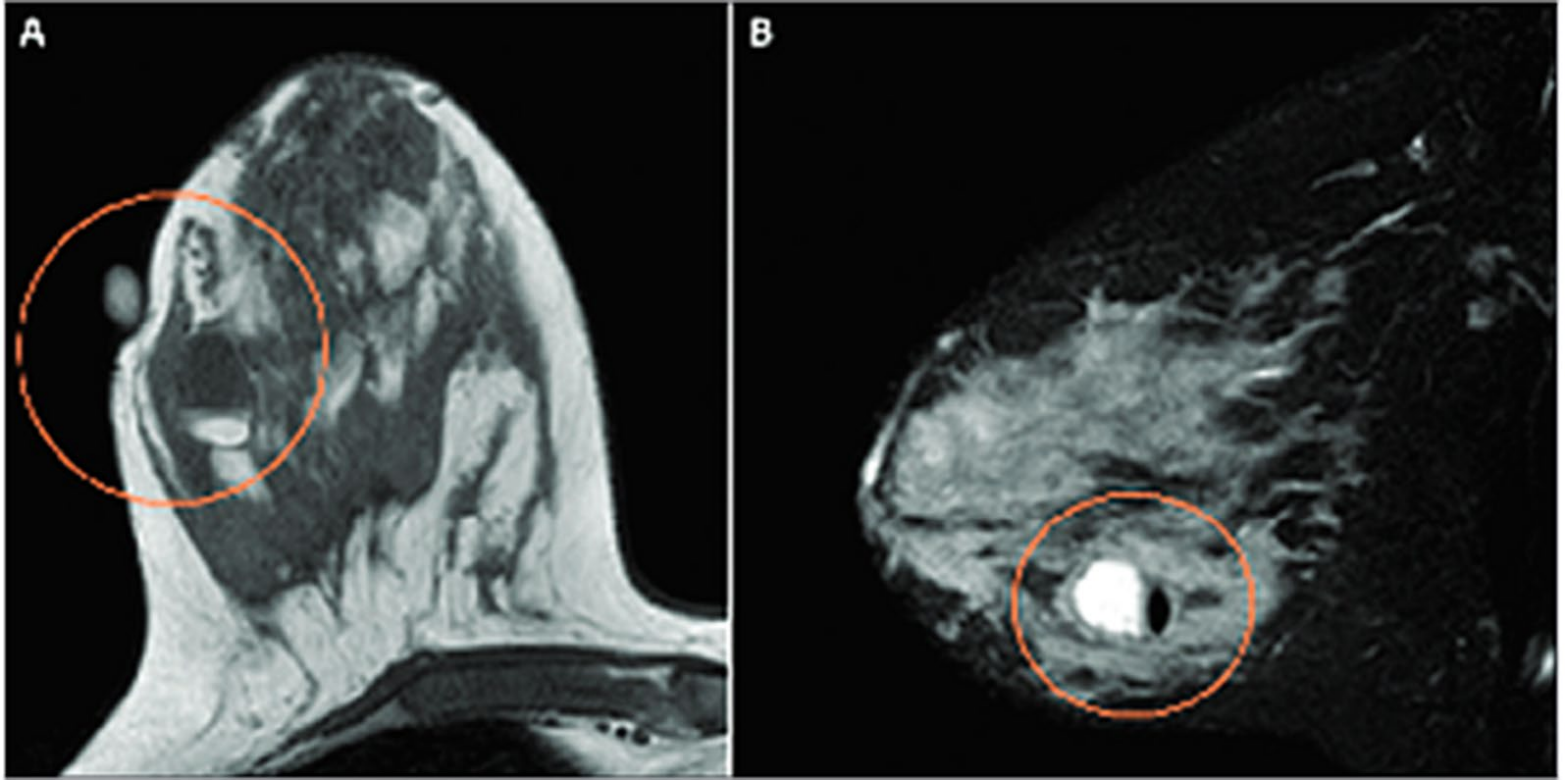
Galactocele. Non Fat-suppressed axial T1-weighted (panel A) and sagittal fat-suppressed T2-weighted (panel B) images of lactating patient with galactocele are presented. *Note*: a cyst with thin septa, heterogeneous content, and fat-fluid level is exhibited and compatible with galactocele diagnosis. Reproduced with permission from Radiologia Brasileira  [19]
